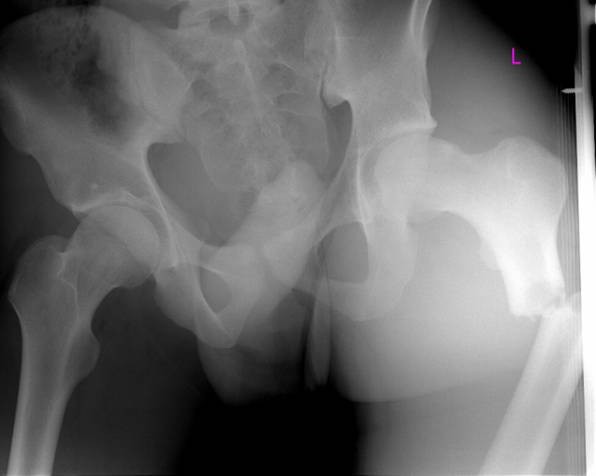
Pre-operative radiograph of the pelvis showing proximal fracture of the left femoral shaft.
